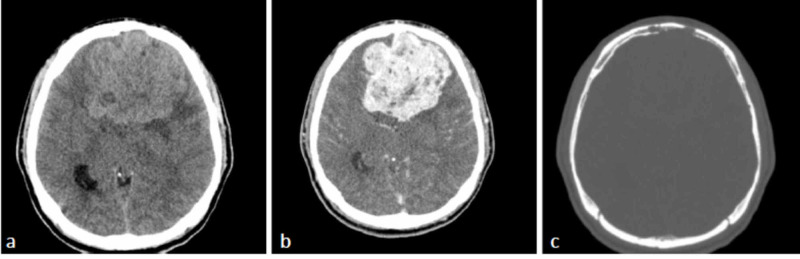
Axial CT scan (a) pre-contrast axial image shows a large heterogeneous mass occupying the basifrontal region spanning across the midline. The mass is hyper-attenuated to the adjacent grey matter. (b) Post-contrast image shows avid enhancement of the mass with non-enhancing necrotic foci. (c) Bone window image shows erosion of the inner endplate of the left frontal bone. CT: computed tomography
